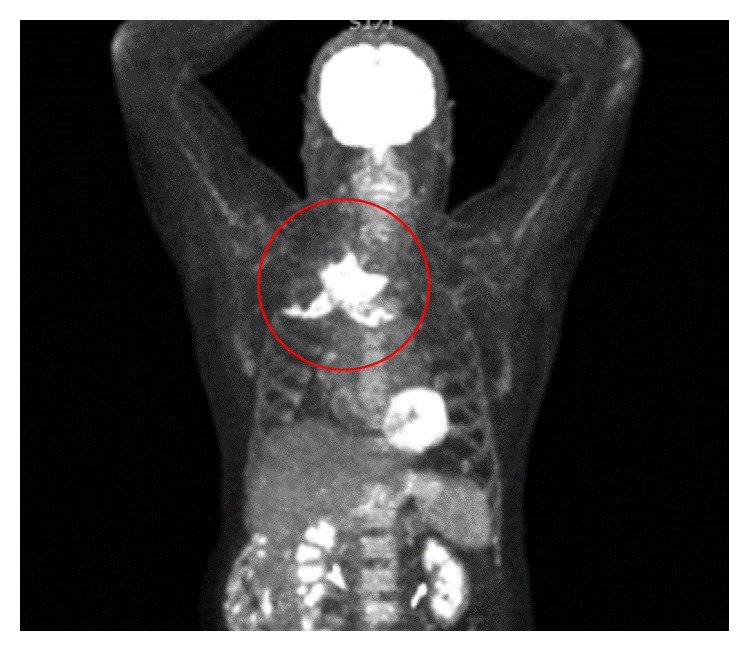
Patient 2. PET-CT frontal view, with reaction in the right sternoclavicular joint.
